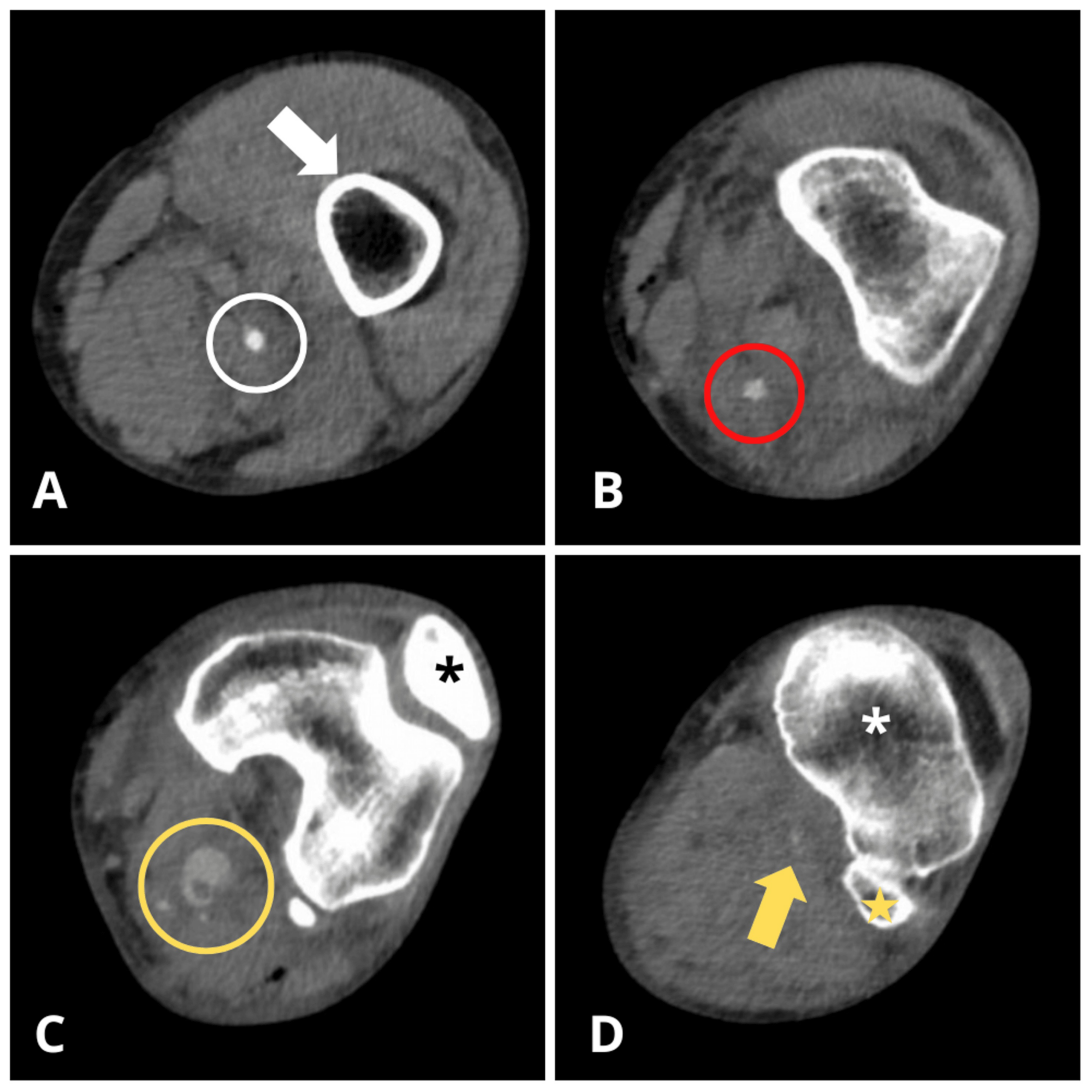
Contrast-enhanced axial CT images of the left leg showing the anatomical course and pathology of the popliteal artery (A) Origin of the left popliteal artery (white circle) as a continuation of the superficial femoral artery after passing through the adductor hiatus, coursing along the posteromedial surface of the distal femoral epiphysis (white arrow). (B) Left popliteal artery in its P1 segment (red circle), demonstrating a course posterior to the supracondylar portion of the femur, extending from the passage through the adductor hiatus to the superior border of the patella. (C) Left popliteal artery in its P2 segment, following an intercondylar retropatellar course as it passes posterior to the knee joint. A saccular image consistent with a pseudoaneurysm (yellow circle) is also visualized at the same axial level as the patella (black asterisk). (D) Left popliteal artery in its P3 segment at the level of the proximal tibial (white asterisk) and fibular (yellow star) articular condyles, demonstrating a marked reduction in blood flow, as evidenced by relative hypodensity of the vessel (yellow arrow) compared to the more proximal segments. CT: computed tomography
